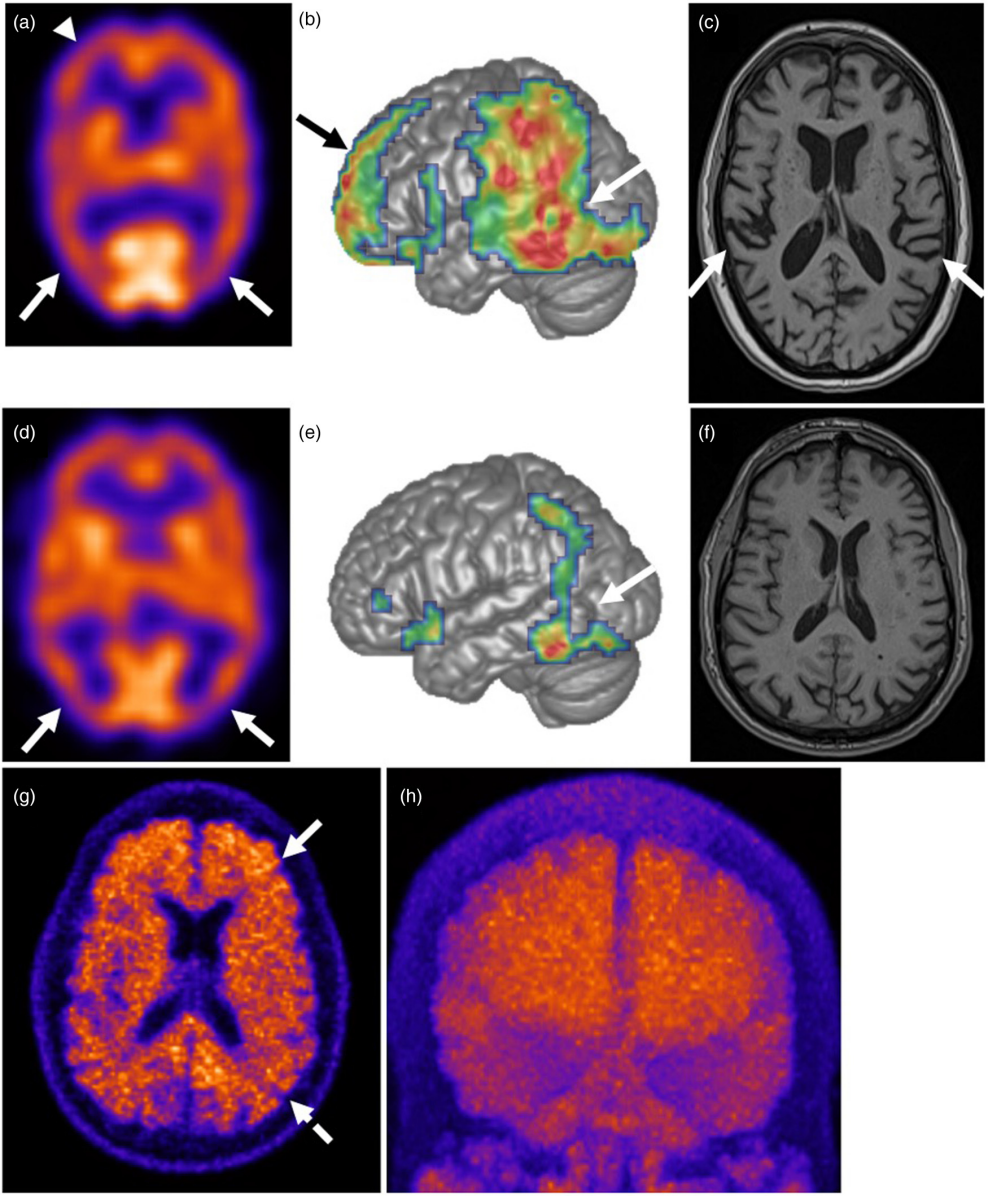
Patient A: Selected transaxial slice of a ^99m^Tc-ethyl cysteinate dimer (ECD) brain perfusion study (a) shows more extensive areas of moderately reduced perfusion to the parietotemporal regions (white arrows) and reduced uptake in the prefrontal region (arrowhead) with relative sparing of the somesthetic and motor cortices and occipital lobes, also shown on statistical parametric mapping (SPM) (white and black arrows, respectively) (b). MRI T1-weighted transaxial images (c) show mild temporoparietal lobe-predominant volume loss (white arrows). Patient B: Selected transaxial slice of a ^99m^Tc-ECD brain perfusion study (d) shows mildly reduced perfusion to the parietotemporal association regions (white arrows), also demonstrated on SPM (white arrow) (e). MRI T1-weighted transaxial images (f) show no regional or global cortical atrophy. On ^18^F-florbetaben (Neuraceq) transaxial PET imaging (g), there is a diffuse tracer localization that shows homogeneous uptake across both the white and gray matter in the frontal and parietal lobes (white solid and dashed arrows, respectively), indicating high amyloid-β deposition. Anterior volumetric maximum intensity projection (h) shows the global extent of tracer uptake seen throughout the cerebral gray and white matter.
